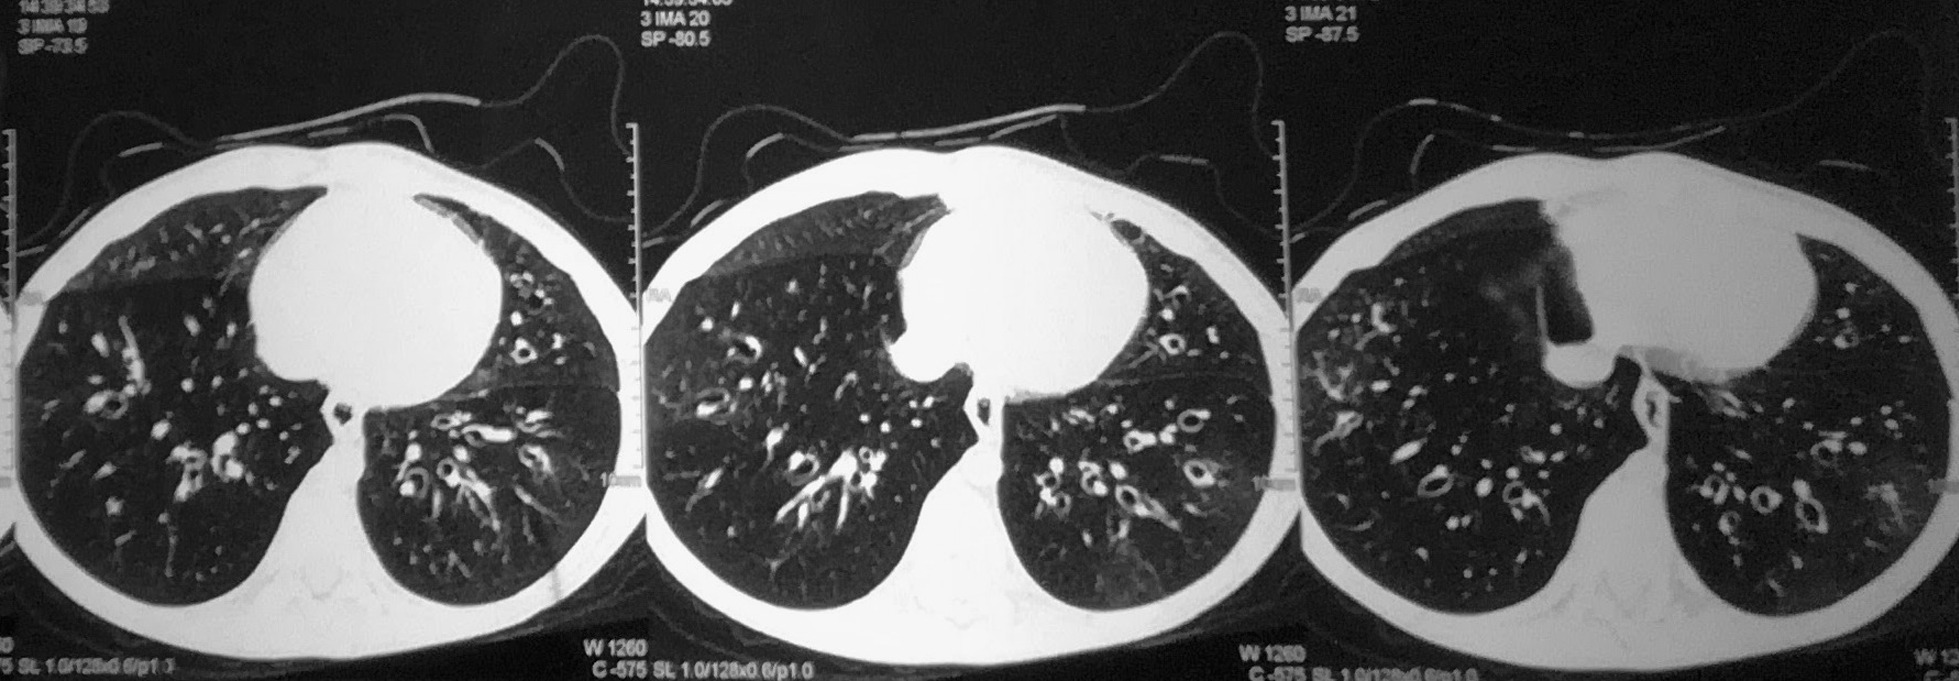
High resolution computerised tomography (HRCT) scan showing bronchiectatic changes in bilateral lower lobes
